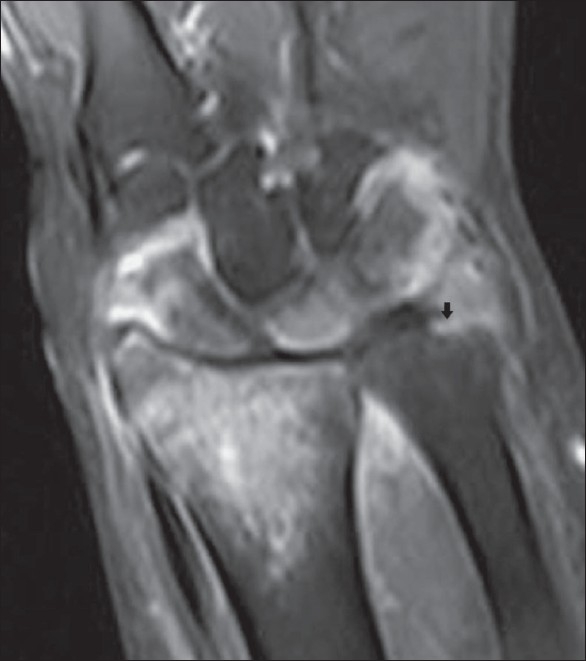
Tuberculous arthritis of the wrist. Coronal, fat-suppressed T2W MRI shows hyperintense bone marrow edema involving the distal radius, scaphoid, lunate and triquetrum. Note increased joint fluid in the distal radioulnar and radiocarpal joints and erosion of the distal ulna (black arrow)
